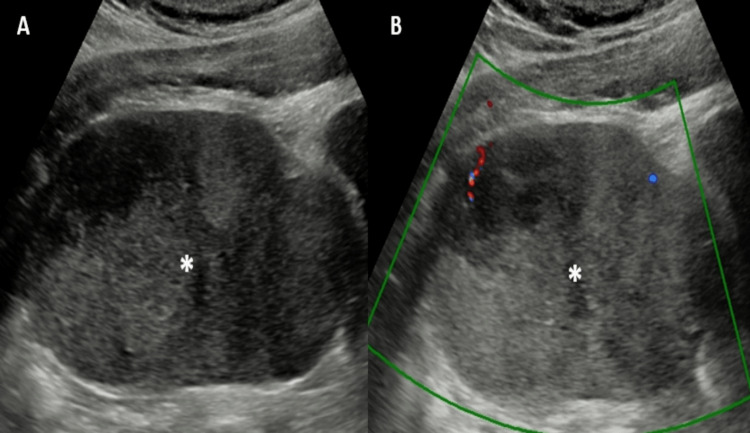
Ultrasound and color Doppler of the abdomen A: Ultrasound shows a well-defined iso-hyperechoic solid mass (asterisk) in the left hypogastric region. Multiple anechoic areas within the mass represent necrotic changes. B: On color Doppler, the mass shows minimal peripheral vascularity.
